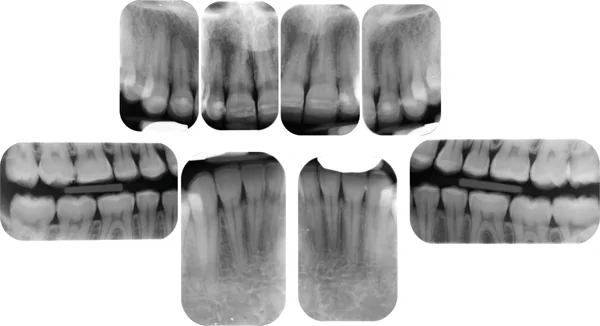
Final radiographic evaluation. Residual cement excesses were identified and subsequently removed following radiographic assessment.
